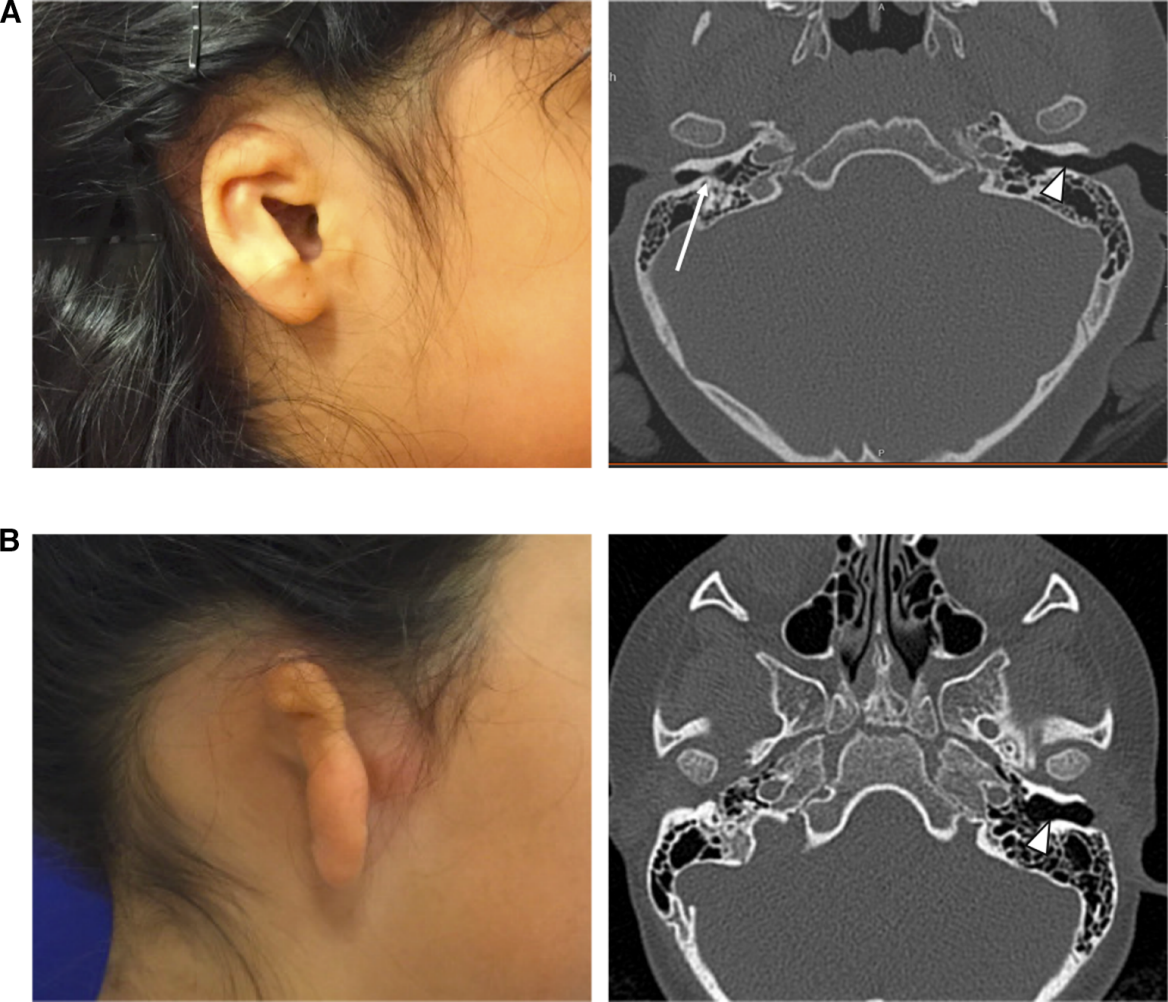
(A) Child with visible canal opening on the right, but severe bony external canal stenosis demonstrated on non-contrast CT scan (long white arrow). Normal canal size is noted on the contralateral side (short white arrow). (B) Child with complete atresia on the right, demonstrated on non-contrast CT scan. Normal canal size is noted on the contralateral side (short white arrow).
